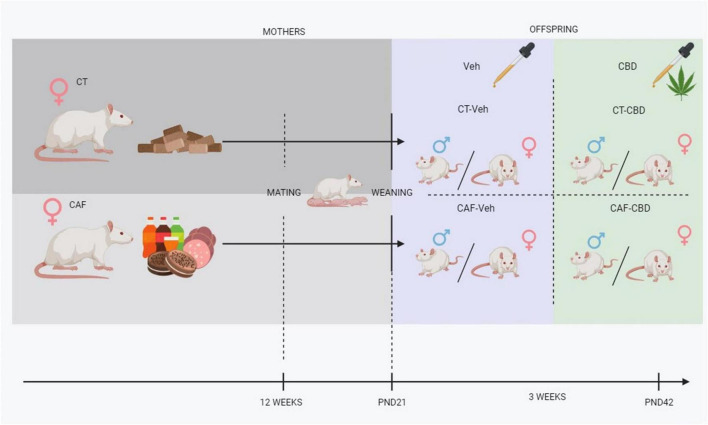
Experimental design. CT, control chow-fed dam; CAF, cafeteria diet-fed dam; Veh, offspring treated with vehicle (corn oil); CBD, offspring treated with cannabidiol (50 mg/kg); PND, post-natal day.
